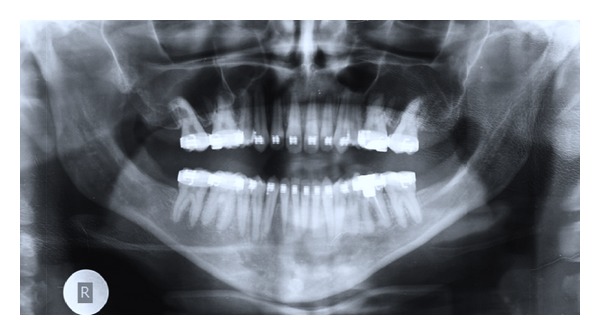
Presurgical orthopantomogram (OPG).
